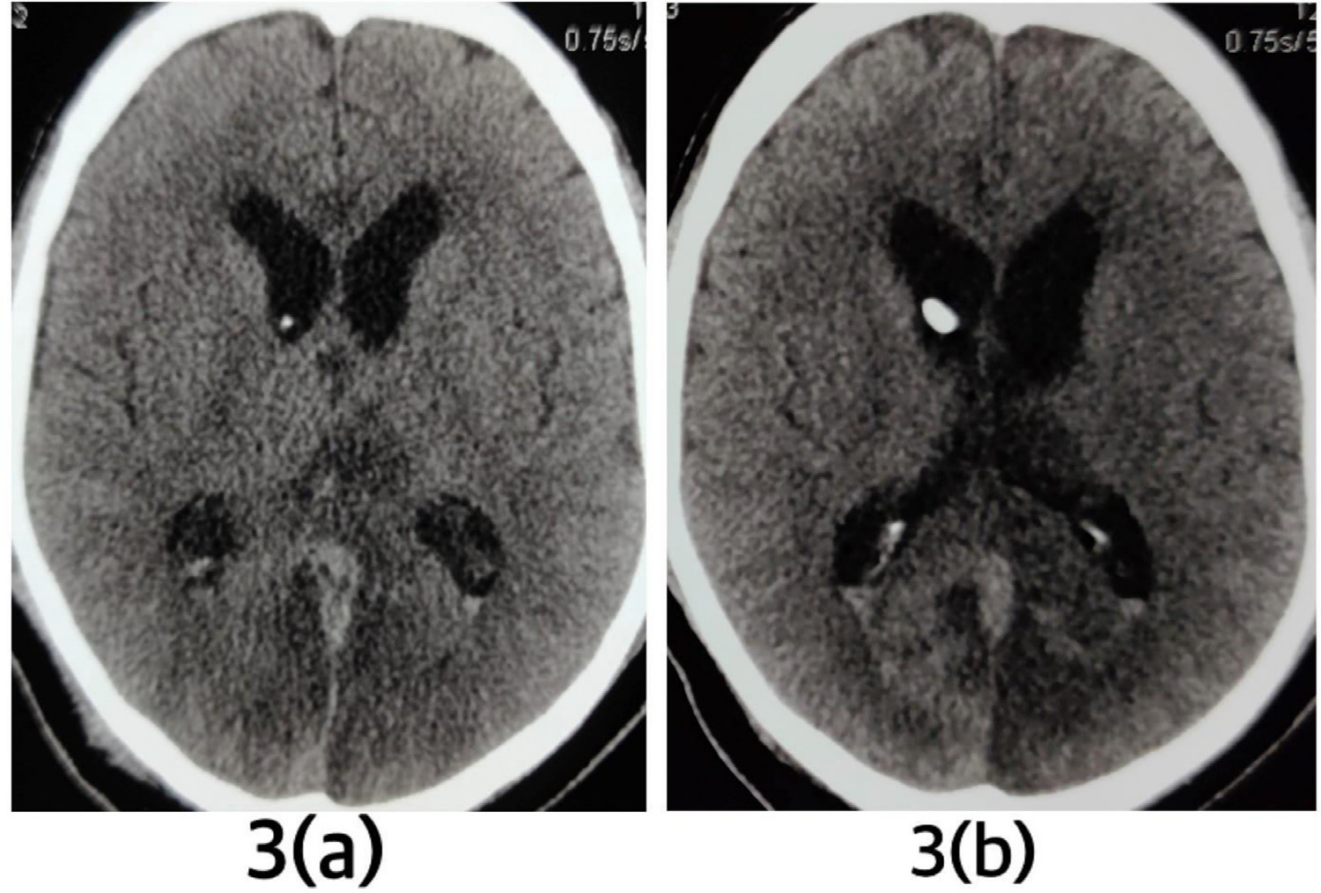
Plain CT of head after endoscopic resection of CC and septal fenestration. (A) There is absence of colloid cyst in third ventricle. (B) An external ventricular drainage is seen in situ and the ventricular system is decompressed.
